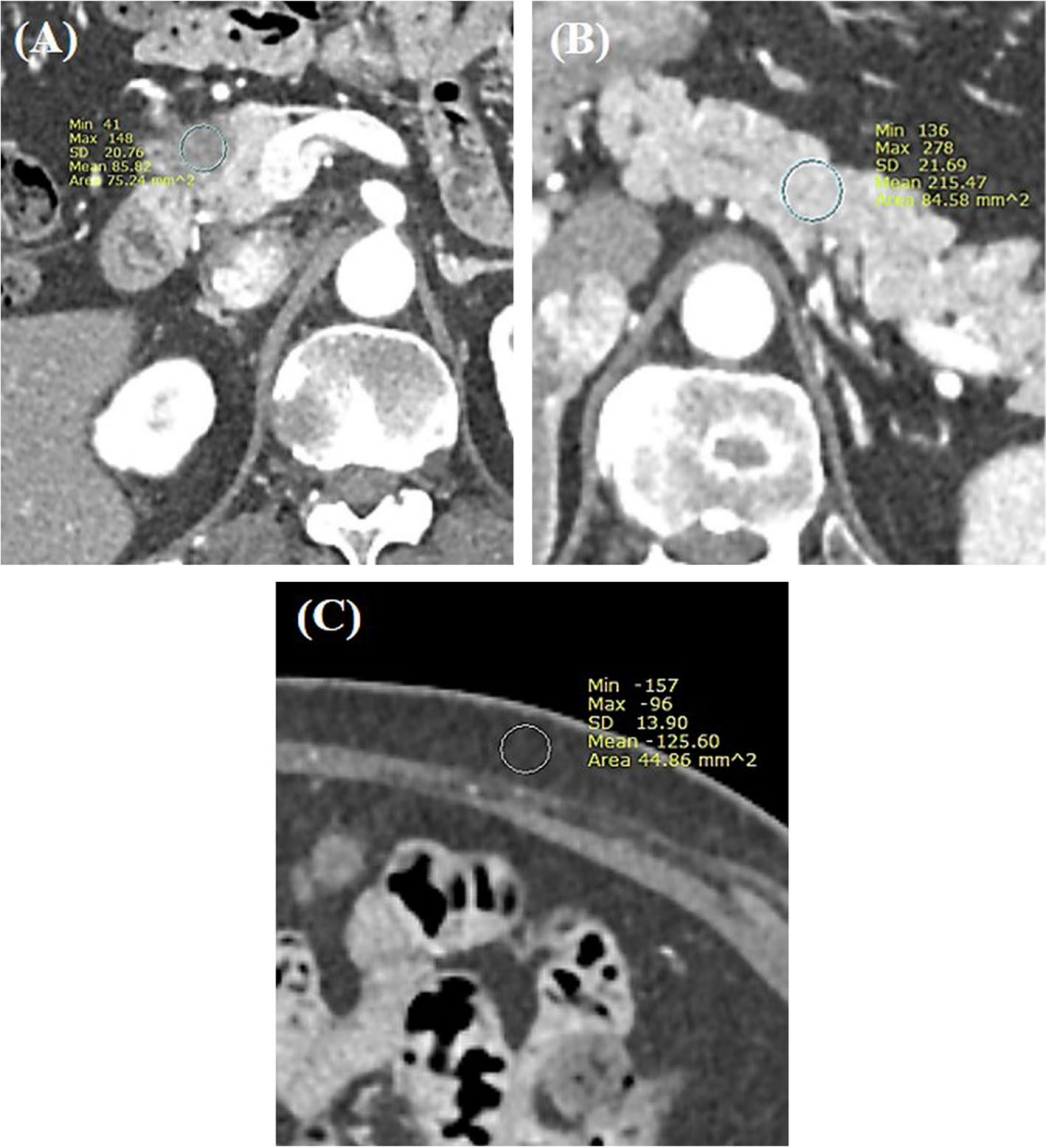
Assessment of CNR for pancreatic ductal adenocarcinoma in a 56-year-old female patient. A An arterial phase axial contrast-enhanced CT image from the high-resolution protocol reveals a 2 cm hypo-attenuating lesion with a mean Hounsfield Unit (HU) value of 85.8. B The mean HU value for the pancreatic parenchyma was 215.47. C Noise level, characterized by the mean standard deviation of subcutaneous fat attenuation, is measured at three distinct locations (one shown here), yielding a value of 13.5. From these measurements, the CNR for the pancreatic ductal adenocarcinoma is calculated to be 9.6
